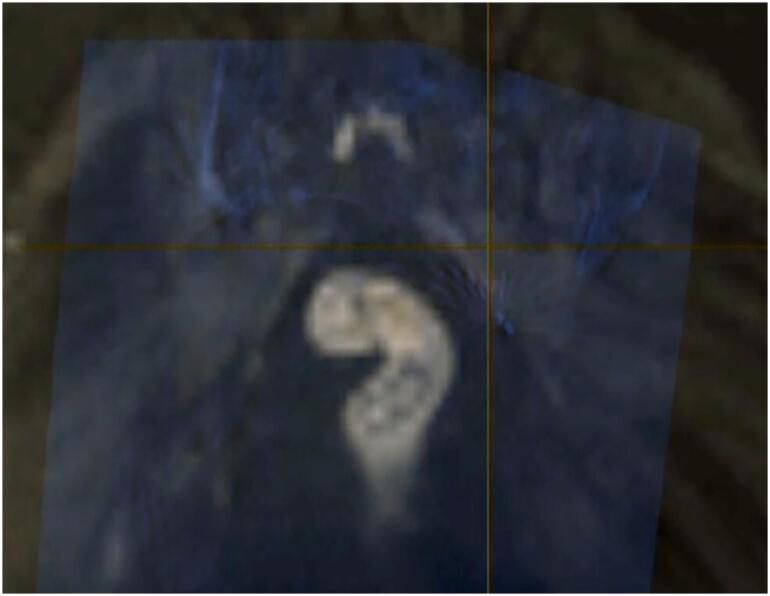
Electrode poles 0 and 1 demonstrated alongside the third sacral nerve (S3) in the coronal reconstruction (MRI image fusion).
